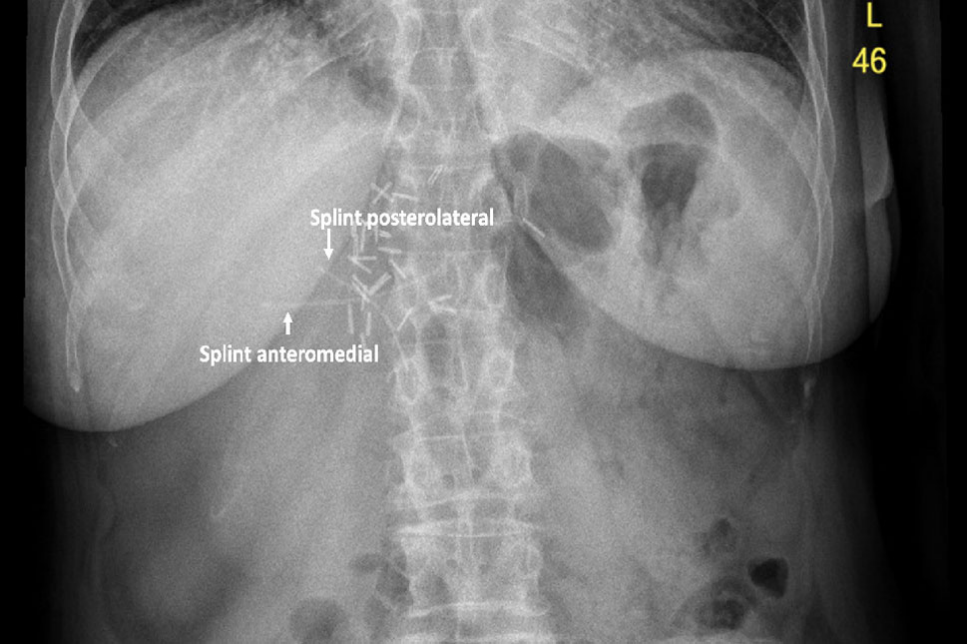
Internally draining biliary splints are placed by abdomen X-ray for visualization 3 weeks after surgery. Internal drainages, placed during hepaticojejunostomy, are released and excreted per via naturalis
